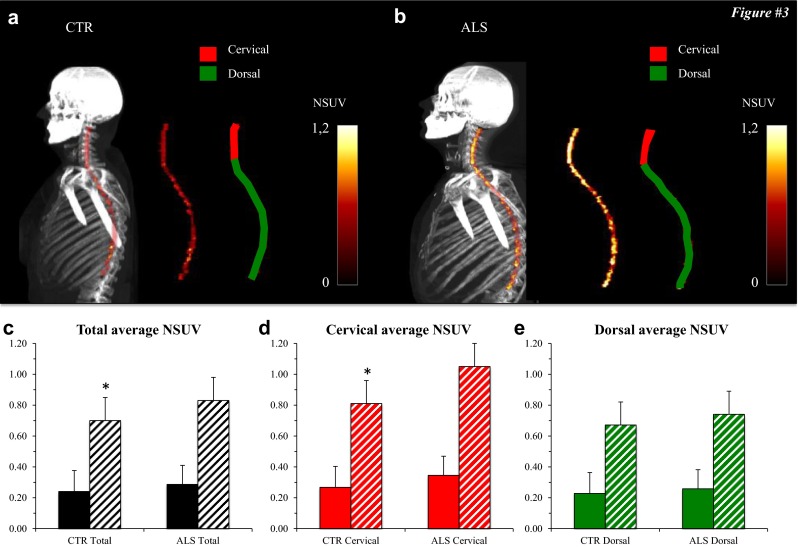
a, b Whole-body maximum intensity projection CT images coregistered with the extracted PET data for the spinal cord in a control subject (a
